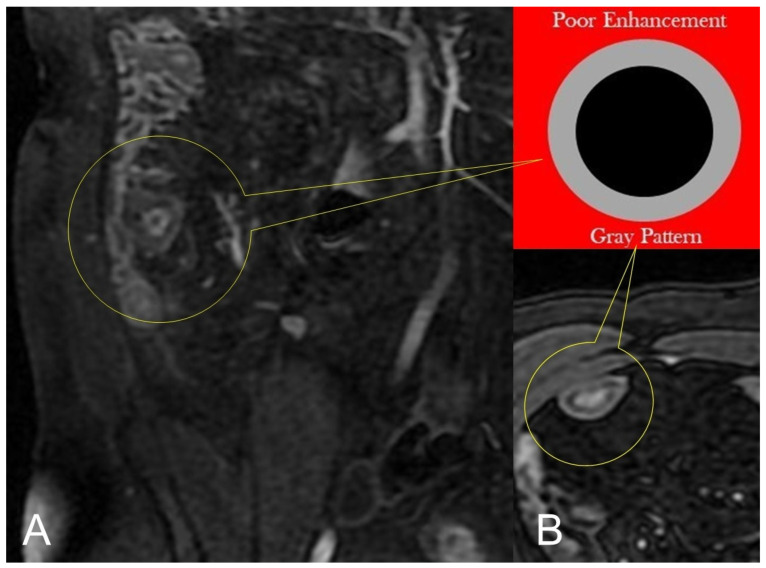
Lack of layered or homogeneous enhancement can be related to fat ((B), axial FIESTA) image, or fibrosis parietal deposition, with poor enhancement and gray pattern on contrast-enhanced fat-suppressed T1-weighted image ((A), coronal image).
